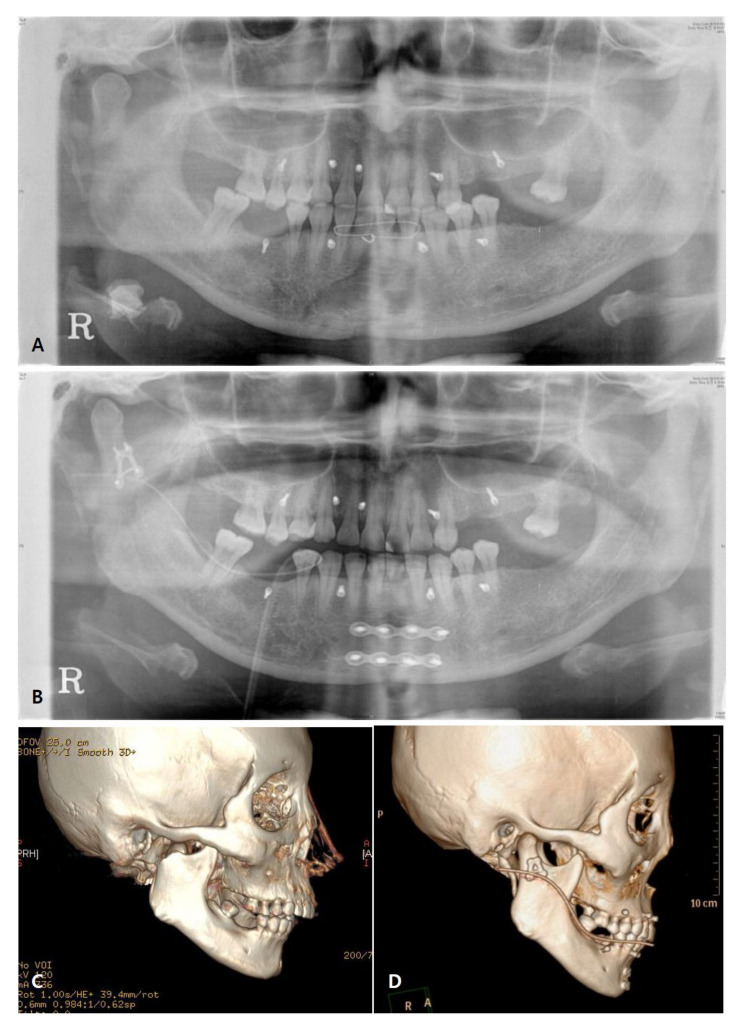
X-ray and 3D facial CT view. (A) Pre-operative panoramic view. (B) Post-operative panoramic view. (C) Pre-operative 3D facial computed tomography scan. (D) Post-operative 3D facial computed tomography scan.
